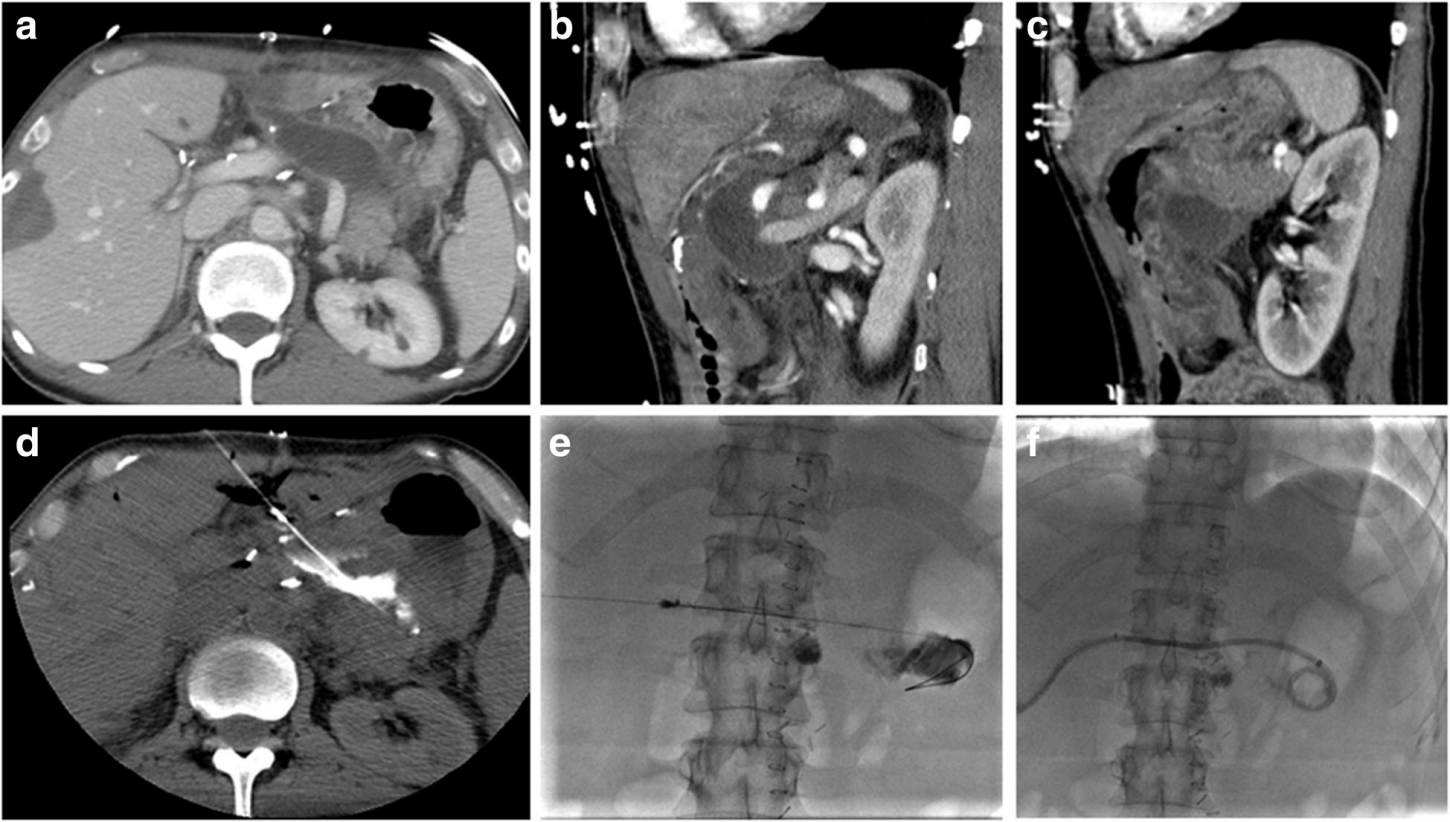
Example of CT usage during a drainage procedure: The patient was a 59 year old female with a postoperative bleed near the pancreatico-gastric anastomosis following liver wedge resection (a). Access from anterior and lateral approaches was not possible because of the left liver (b) and stomach locations (c). Posterior approach was difficult due to the left kidney and splenic arteries. CT (d) was very useful to guide the needle track in 3 dimensions. Once the target had been reached, the operator switched from CT to angiography (e) to set up the drain (f)
